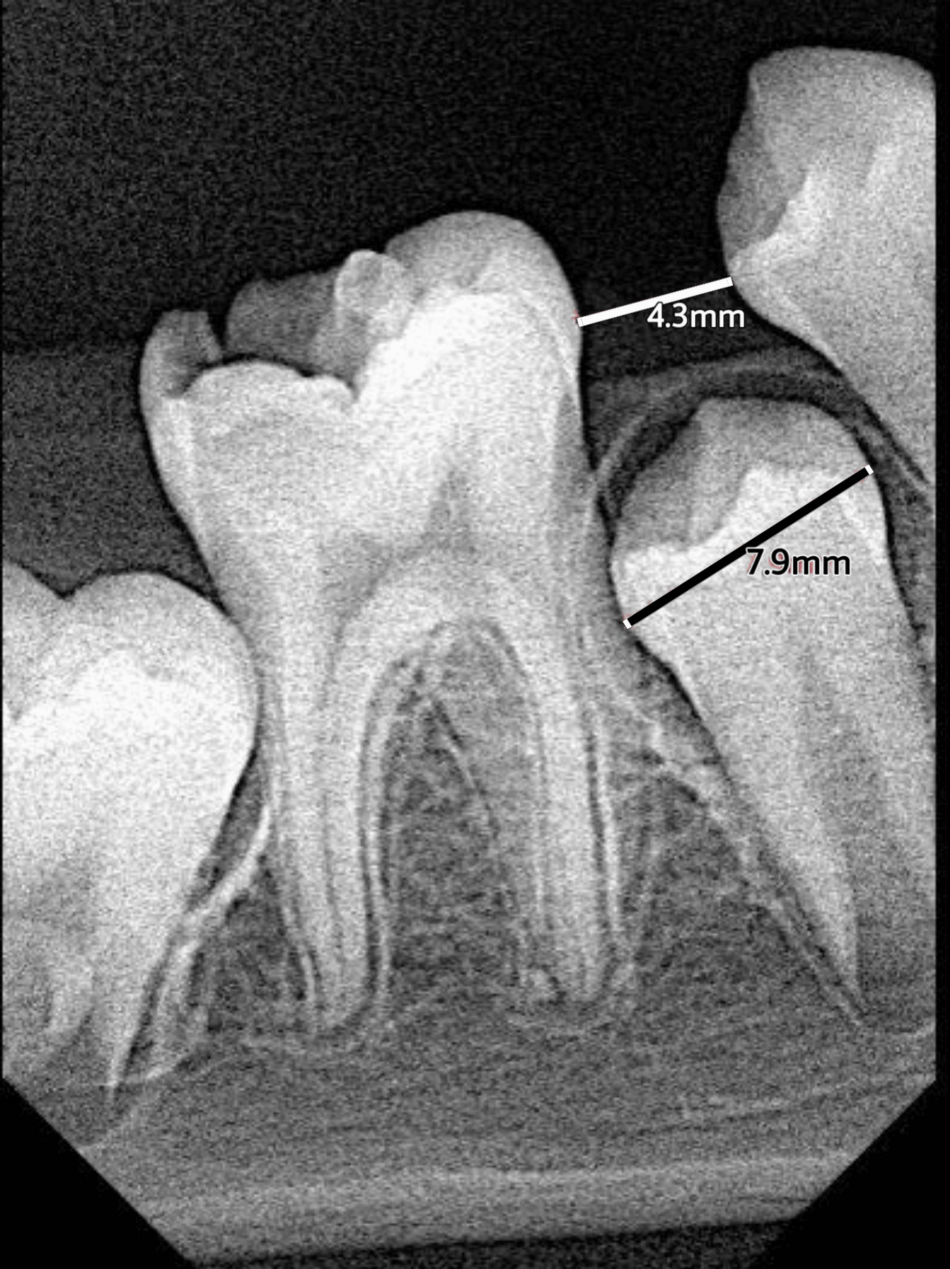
Pre-operative X-ray (available space 4.3 mm)
